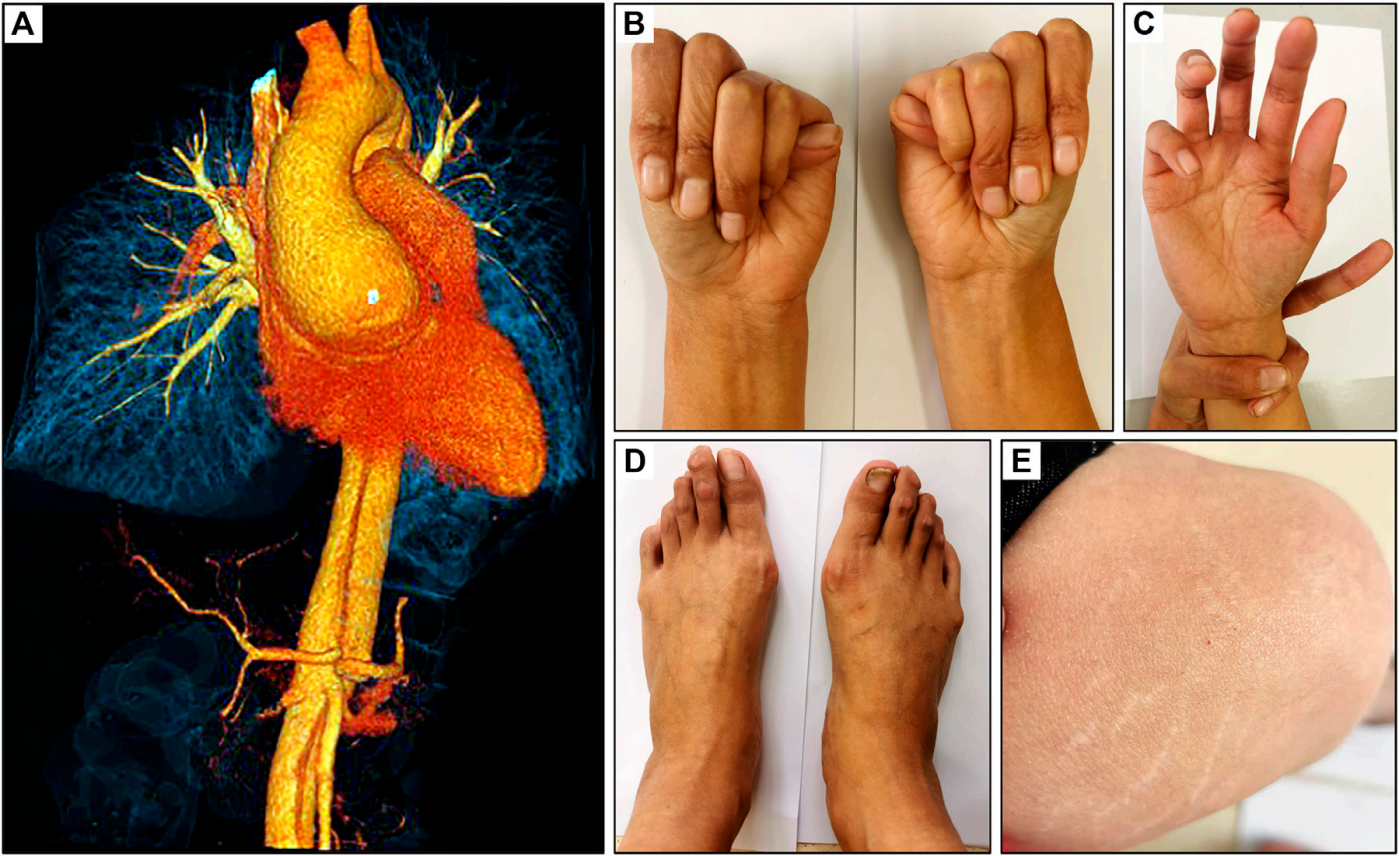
Clinical presentation of a 32-year-old female with MFS. (A) CT scan revealed an aortic root dilatation and Debakey I dissection, (B) positive thumb sign, (C) positive wrist sign, (D) plain pes planus and (E) skin striae.
